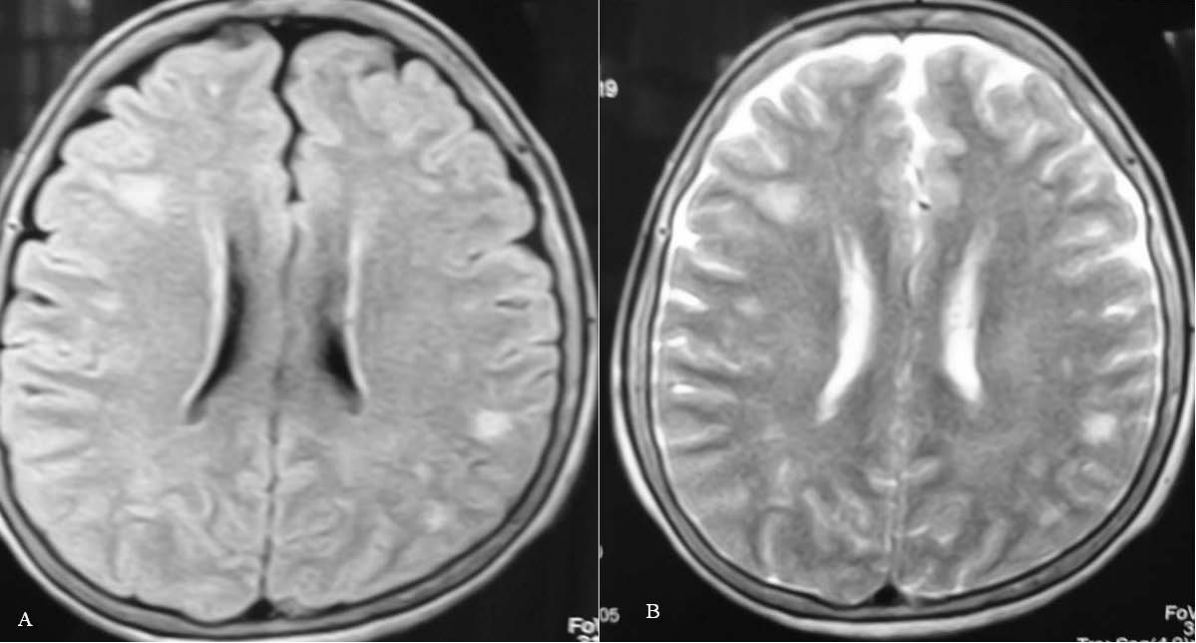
Axial magnetic resonance images (MRI) of the brain demonstrating nodular subcortical lesions suggestive of demyelination in frontal and parietal lobes . A) T1-weighted MRI. B) T2-weighted MRI.
